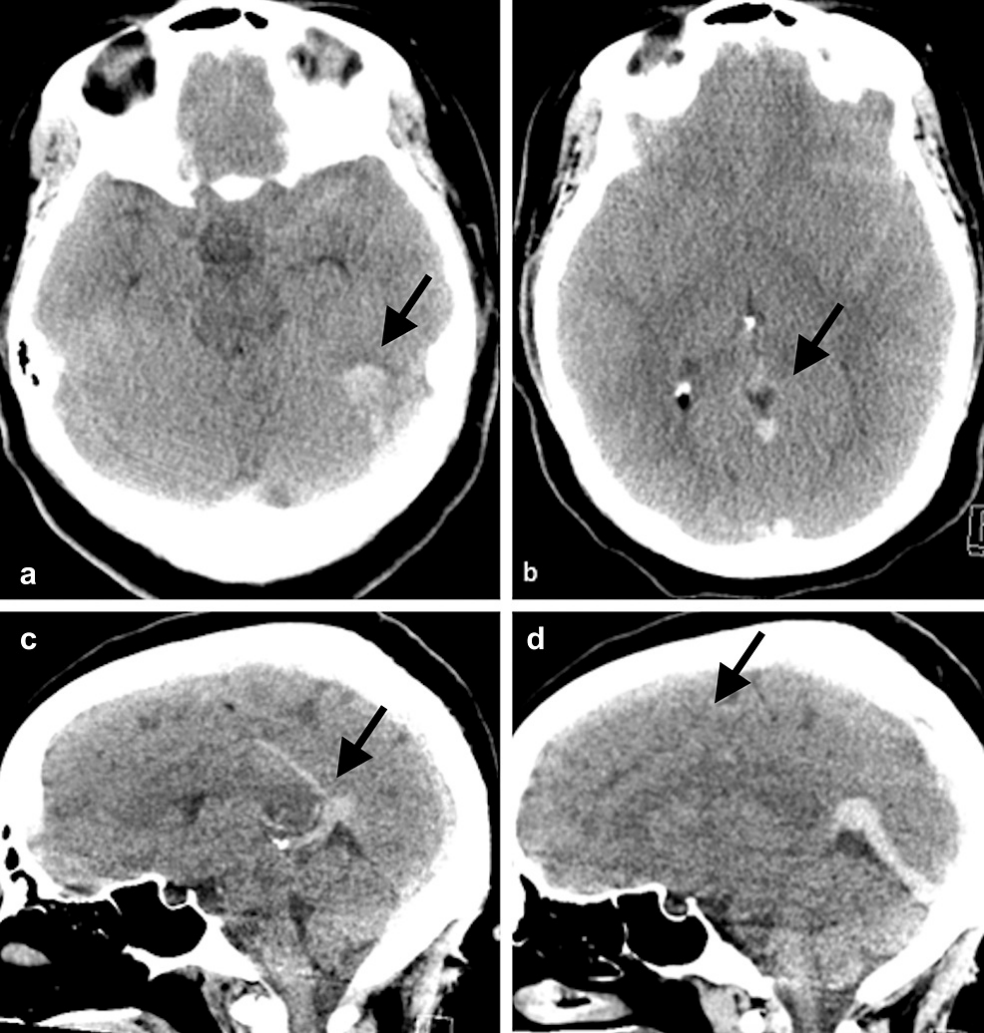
CT head without contrast Small intraparenchymal hematoma in the left temporal lobe (a) and a small amount of subarachnoid hemorrhage in the left cerebral hemisphere (b). Diffuse dural sinuses involving the superior sagittal sinus, transverse sinuses bilaterally, and inferior sagittal sinus (c). Multiple thrombosed cortical veins and evidence of deep venous thrombosis involving the vein of Gallen and straight sinus to the torcula (d). Diffuse sulcal effacement and ventricular compression, in keeping with increased intracranial pressure. There is no transtentorial or tonsillar herniation CT: computed tomography
